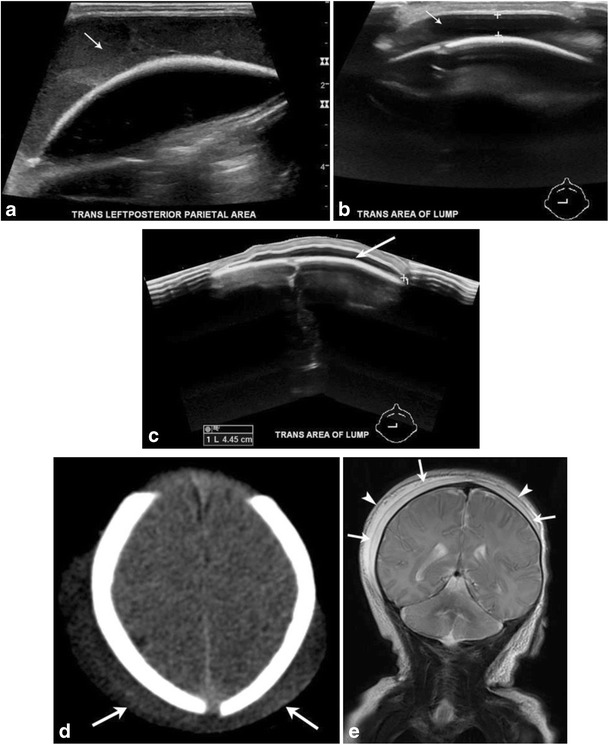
Caput succedaneum and subgaleal hemorrhage Grayscale ultrasound images (a-c) of the scalp in a newborn male demonstrate a fluid collection that crosses midline, is deep to the subcutaneous fat/galeal aponeurosis and superficial to the periosteum/calvarium (noted as thick echogenic interface) consistent with a subgaleal hemorrhage. Axial CT (d) image in a 1-day-old male with history of traumatic delivery demonstrate scalp soft tissue overlying bilateral parietal regions and crossing the sagittal suture (arrows). Follow-up coronal MR image (e) demonstrates a deep subaponeurotic scalp fluid collection crossing the sagittal suture and extending anteriorly into the right temporal region, consistent with subgaleal hematoma (arrows). A more superficial overlying fluid collection with a similar distribution also noted (arrowheads). This collection is within the subcutaneous fibrofatty tissues superficial to galea aponeurosis and is consistent with caput succedaneum
